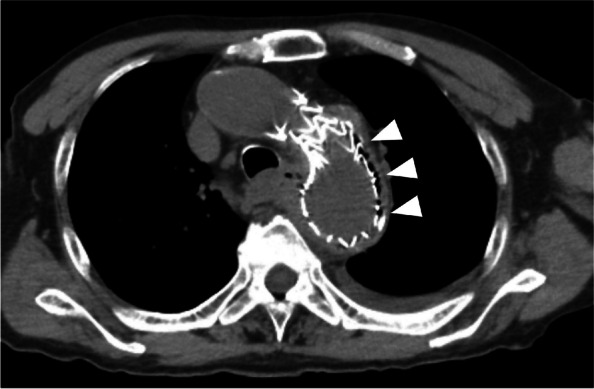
Chest CT findings. Fluid collection and air bubbles were observed around the aortic stent graft (arrowheads)
